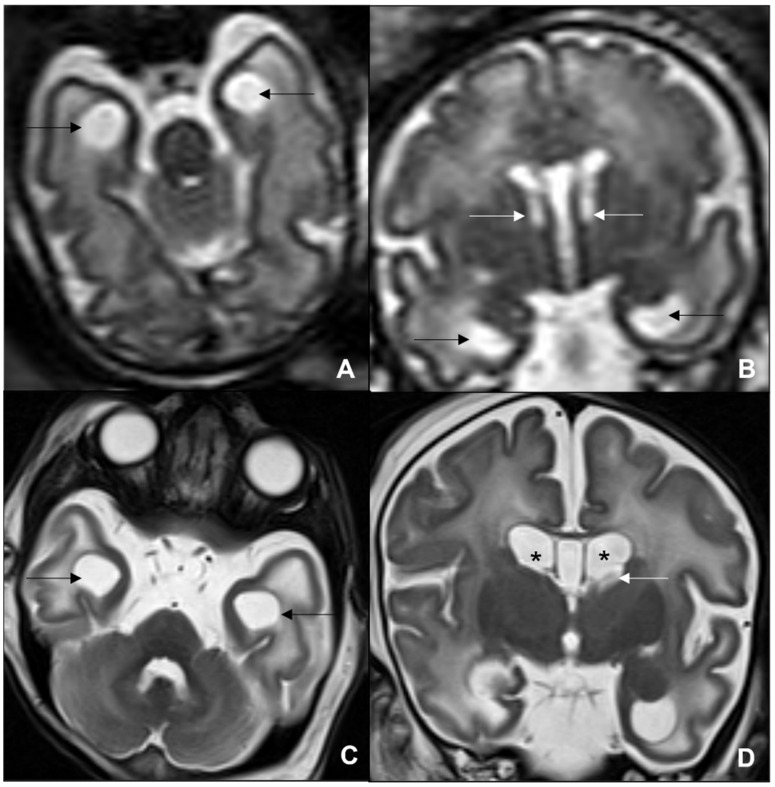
Fetal MRI performed on a fetus affected by Rubella at 27 weeks of gestational age, showing bilateral temporal cysts (black arrows in (A,B)), and subependymal cysts (white arrows in (B)). Neonatal MRI performed on the same patient at six days after birth, showing temporal cysts (black arrows in (C,D)) ventricular dilatation (black asterisks in (C,D)), and subependymal cysts (white arrow in (D)).
